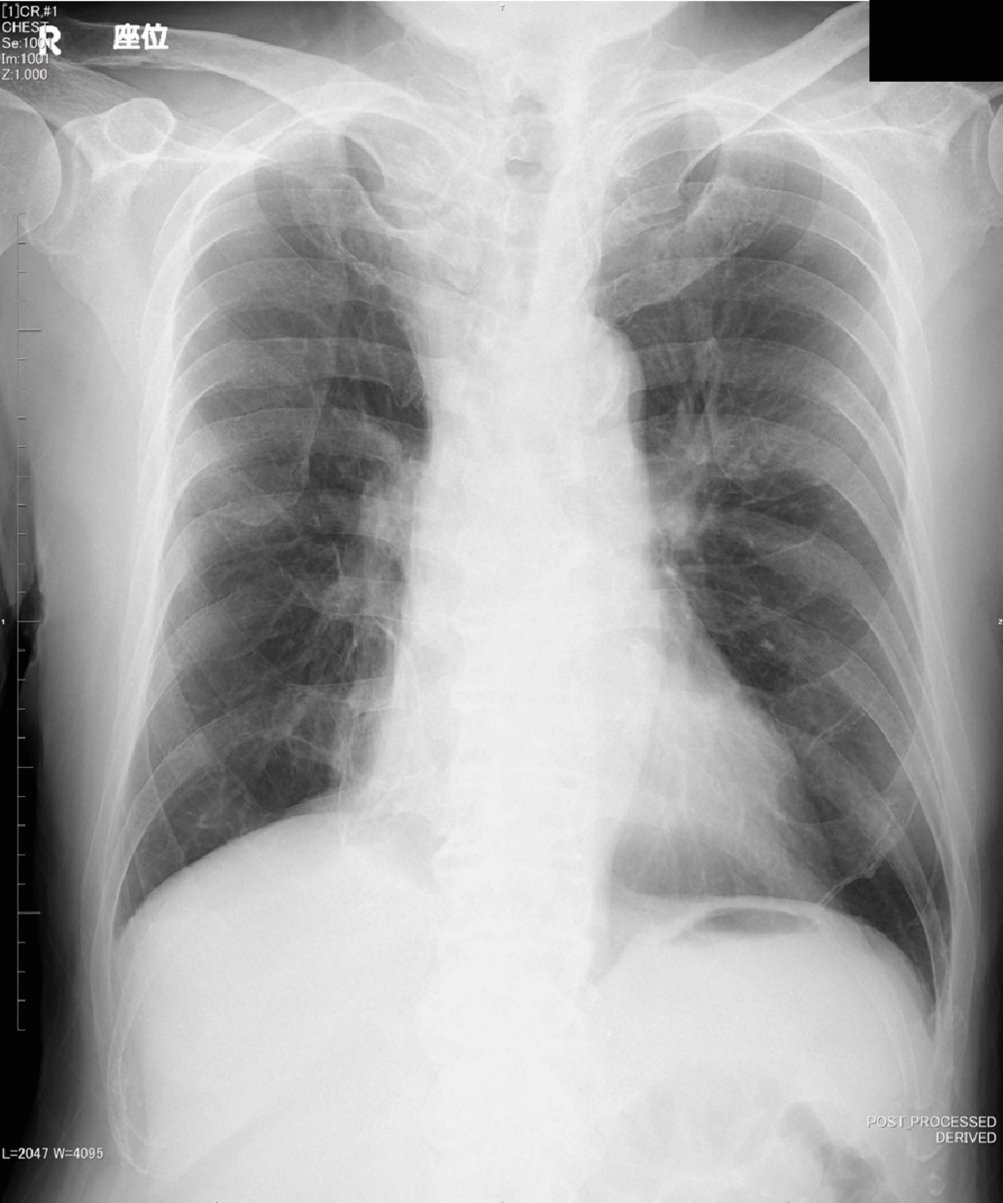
Chest X-ray on day 15. Chest X-ray showing with and without infiltration. No new areas of infiltration was noted.
